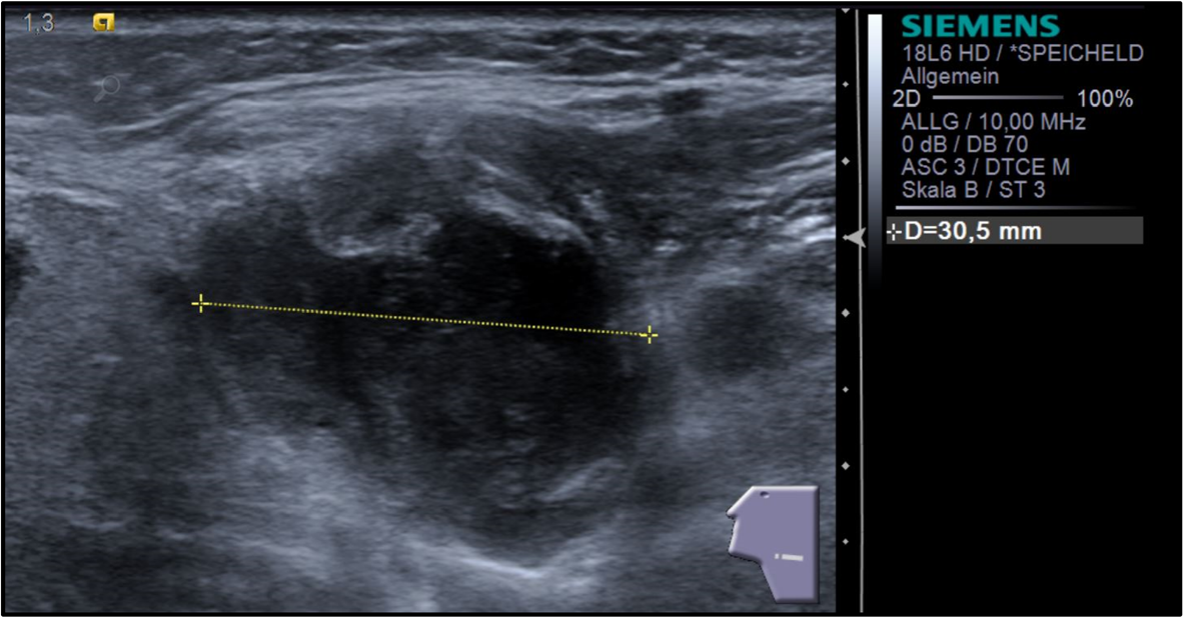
Sonographical image showing the extremely increased lymph nodes 25 days after tooth resection
